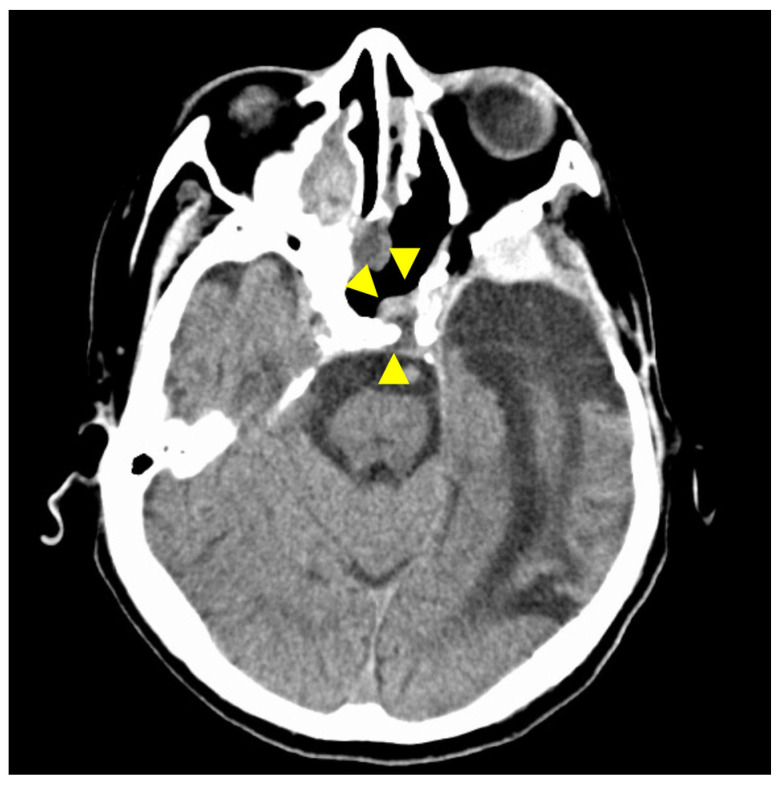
CT findings at 30 months after surgery. The isodense area at the tumor resection site shows no tendency to increase, and there is no evidence of recurrence on CT imaging. Arrowheads indicate the site of tumor removal. The isodensity in the left ethmoid sinus is due to chronic sinusitis.
